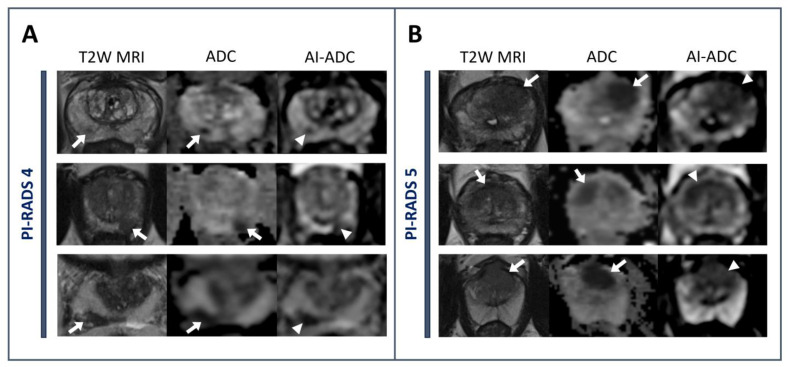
Representative cases from the ProstateX dataset with Prostate Imaging Reporting and Data System (PI-RADS) category 4 and category 5 lesions. PI-RADS category 4 lesions are located in right mid-peripheral zone, left apical peripheral zone, and right mid-base peripheral zone, from top to bottom, respectively (A). Lesions display a homogeneous hypointense signal on T2-weighted MRIs (T2W MRI) and original apparent diffusion coefficient (ADC) maps (arrows) and have a homogenous hypointense appearance on AI-ADC maps (arrowheads), whereas the lesions categorized as PI-RADS category 5 are situated in the left apical–mid anterior transition zone, midline apical–mid anterior transition zone, and midline apical–base anterior transition zone, from top to bottom, respectively (B). These lesions exhibit a hypointense signal on T2W MRI and the original ADC map (arrows) and also demonstrate a hypointense appearance on AI-ADC maps (arrowheads).
